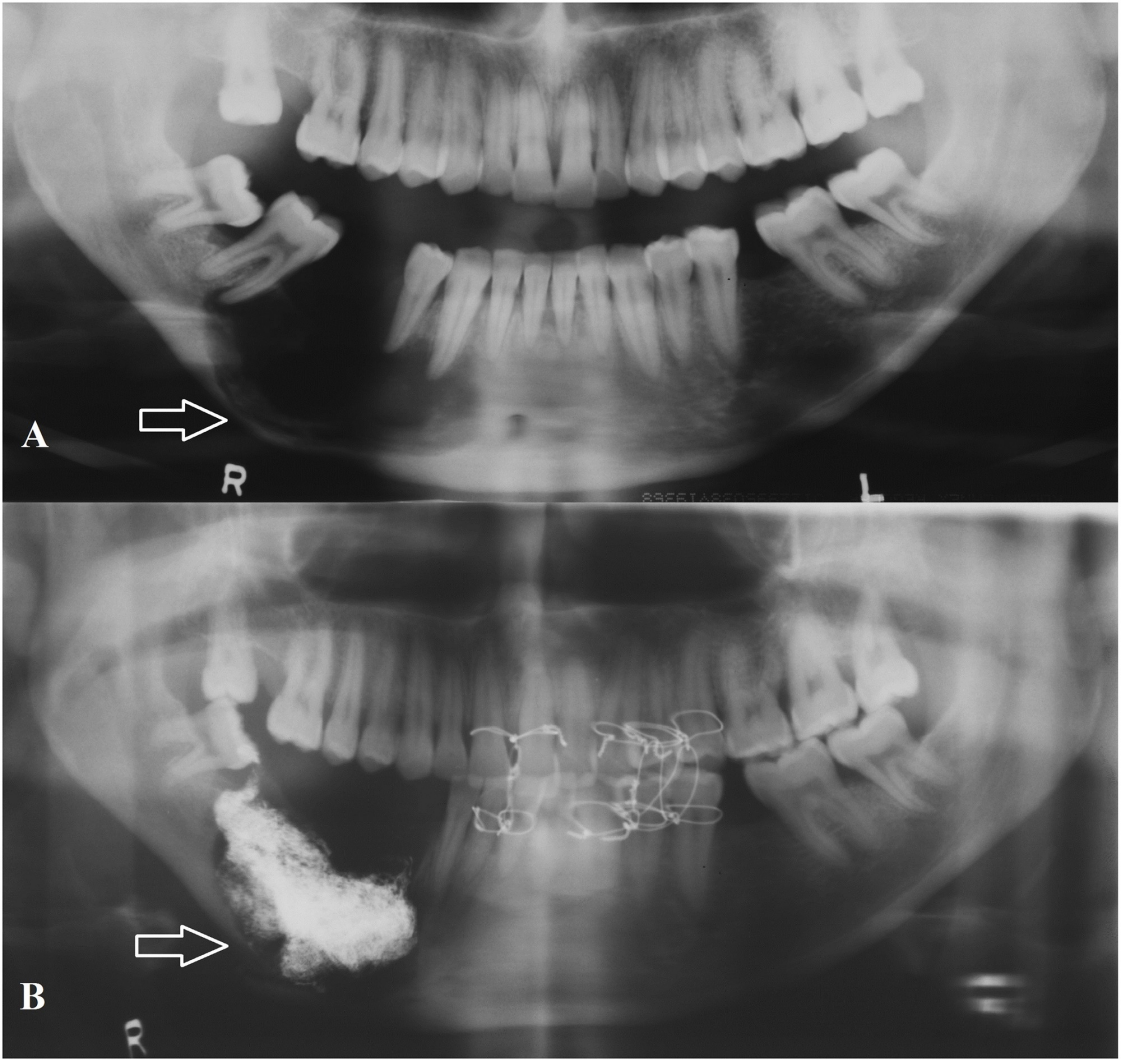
A: Pre-operative panoramic radiograph of residual cyst in the mandible with notable thinning of the mandibular cortex. B: Post-operative panoramic radiograph of same patient showing pathological fracture of mandible with BIPP in situ to prevent post-operative sepsis.
